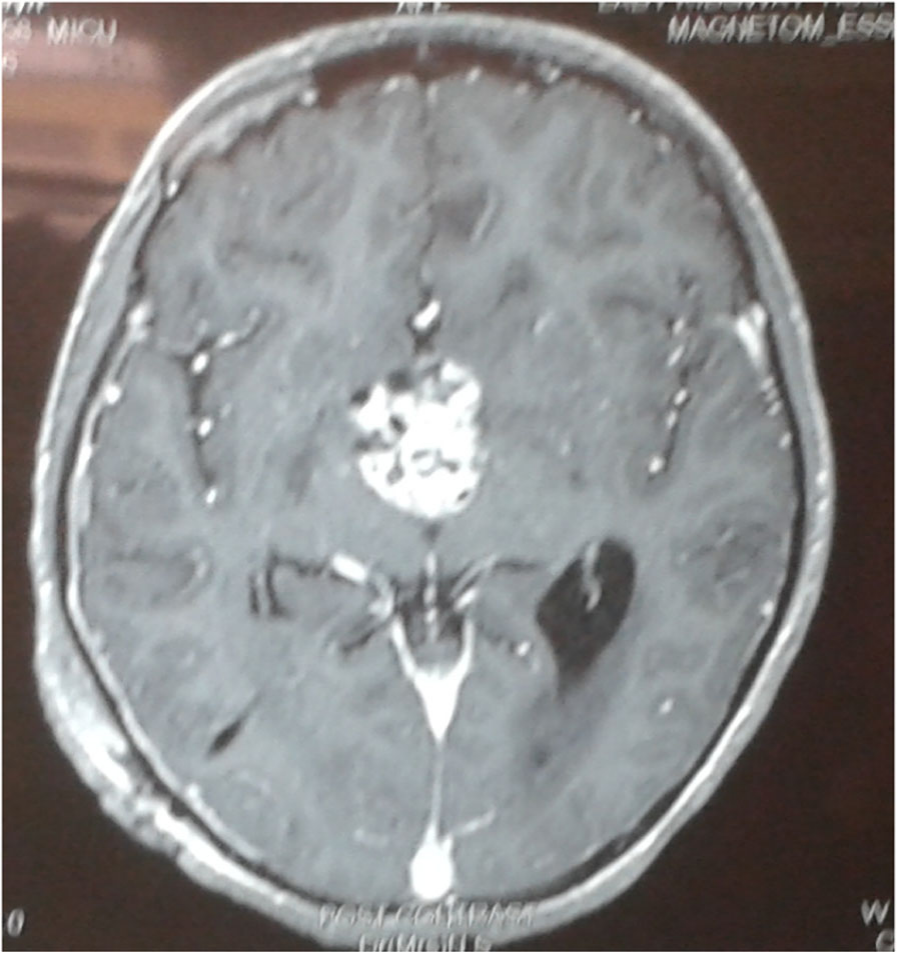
Magnetic resonance imaging post-contrast axial image of the lesion
